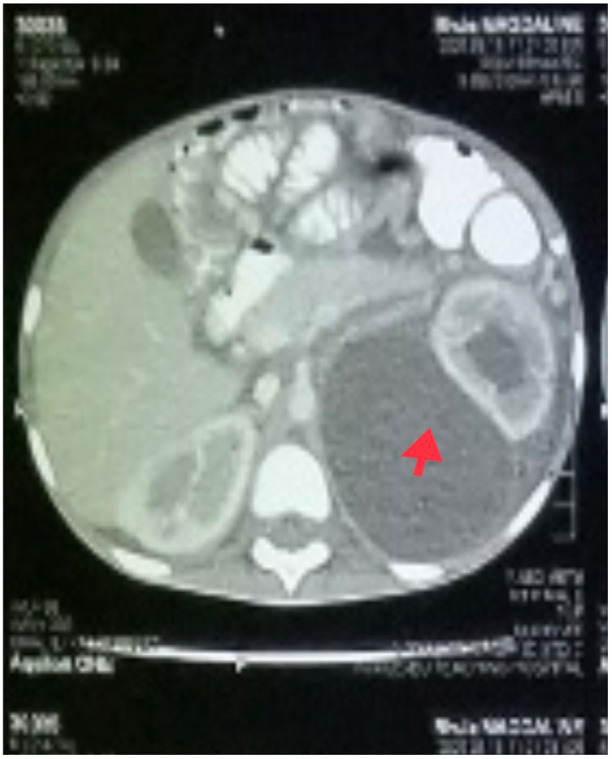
A cross-sectional view of the abdominal CT scan showing large left renal cyst (red arrow) with hydronephrosis.
